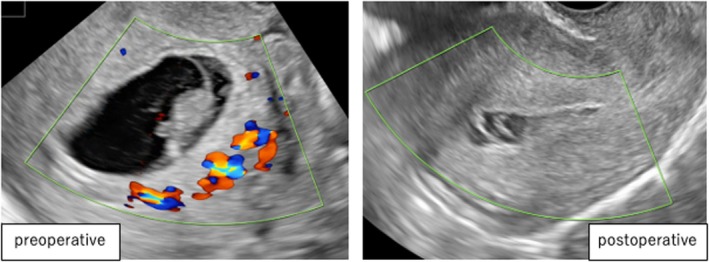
Intraoperative transvaginal ultrasound with color Doppler. Left: Before evacuation, blood flow is detected from the lower part of the image. Right: After evacuation, the intrauterine blood flow has disappeared.
